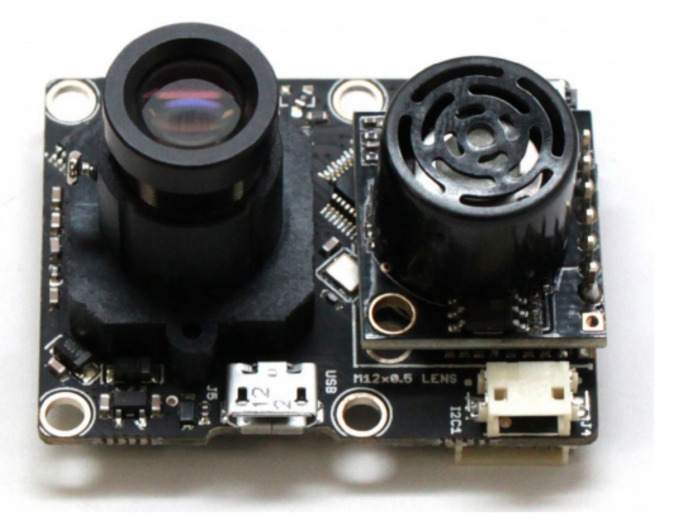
The PX4FLOW optical flow sensor with mounted lens on the left and ultrasonic sensor on the right [93].
